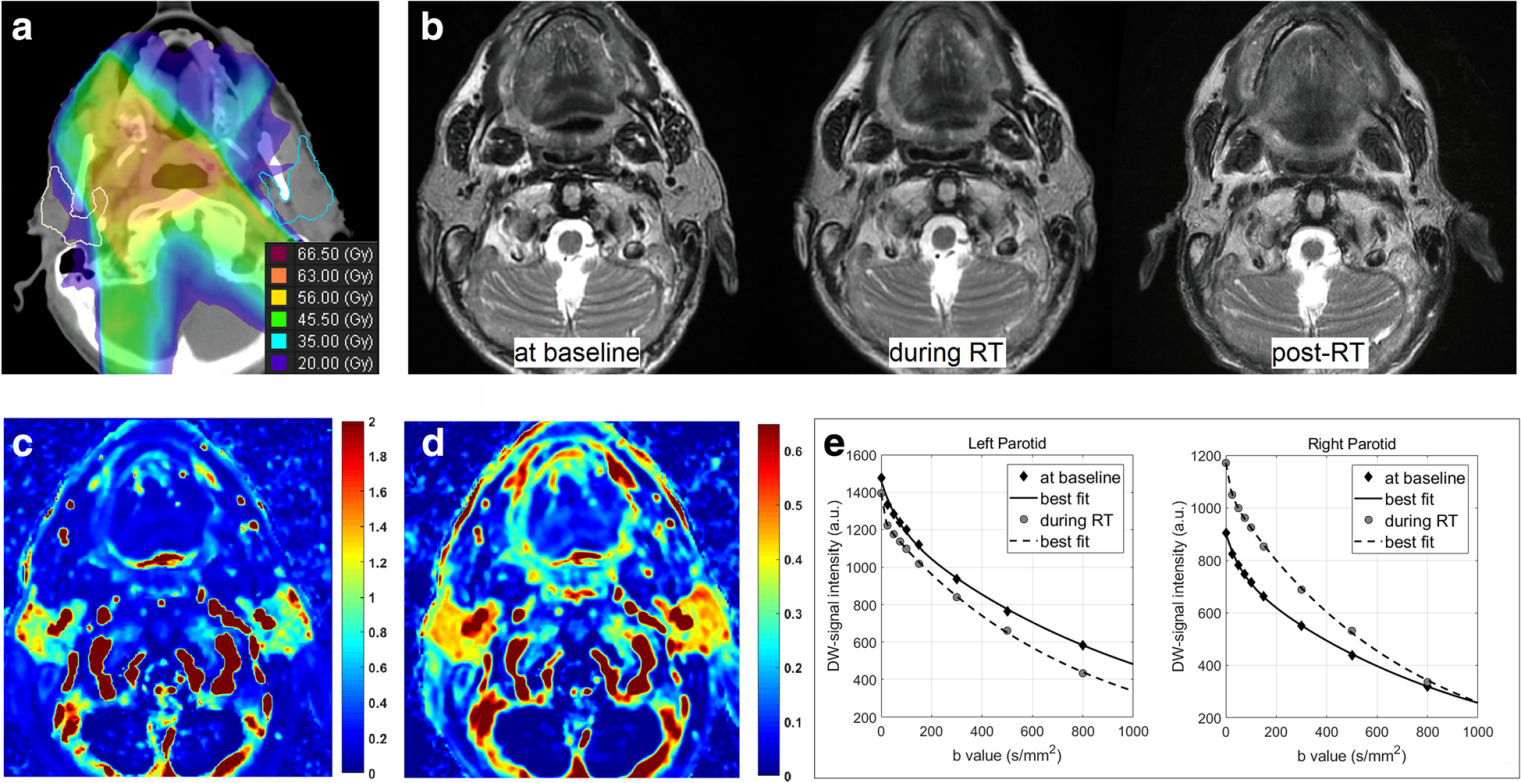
A 68-year-old man affected by a tonsil carcinoma: dose distribution overlaid on the central section of the parotid glands on axial CT (a); T2-weighted images before, at 2 weeks of radiotherapy and 8 weeks after radiotherapy (b) documenting a substantial gland shrinkage; the corresponding maps of K^trans^ in min^− 1^ (c) and IAUGC in a.u. (d), at baseline, indicating well perfused parotids*.* Curves of DW-signal attenuation of parotids at baseline and during RT (e): the modification of the DW-signal attenuation curves during RT documents a noticeable increase in tissue water diffusivity, suggesting a considerable decrease in cell density as a consequence of the radiation damage
